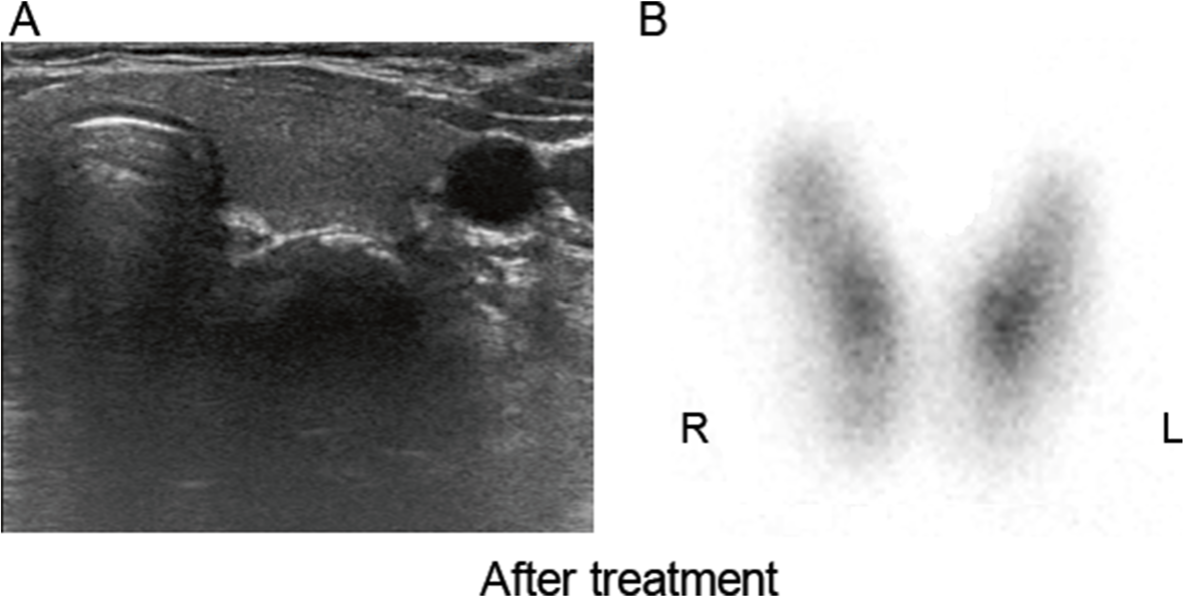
Follow-up ultrasound scanning and 99 m-Technetium scintiscan. A. Follow-up ultrasound scanning demonstrated the disappearance of the hypoechoic nodule in the left thyroid lobe. B. Follow-up scintigraphy showed the disappearance of the “hot” nodule in the left thyroid lobe and an even distribution of radionuclide in both lobes.
